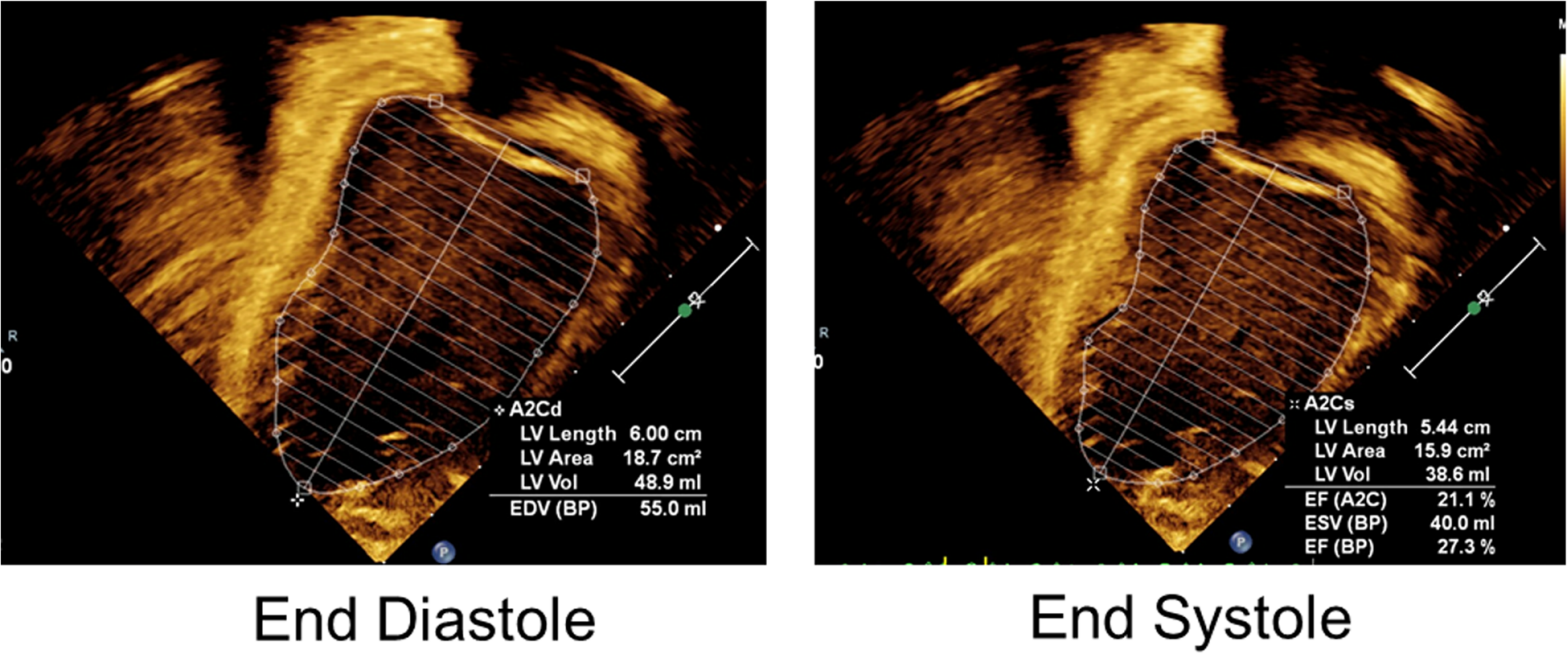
Patient echocardiogram. An apical, left two-chamber view shows moderately dilated left ventricle, mild concentric left ventricular hypertrophy and severely reduced systolic function. Left ventricular end diastolic diameter Z score 5.59 by M-mode and end-diastolic volume by 5/6 area length method is Z score 4.98. left ventricular dp/dt of 1398. There is also a LV diastolic dysfunction with fusion of E and A waves of inflow doppler pattern. There is moderate to severe mitral valve regurgitation and mild to moderate tricuspid regurgitation. The aortic and pulmonary valves are trivially regurgitant. There is no pericardial effusion
